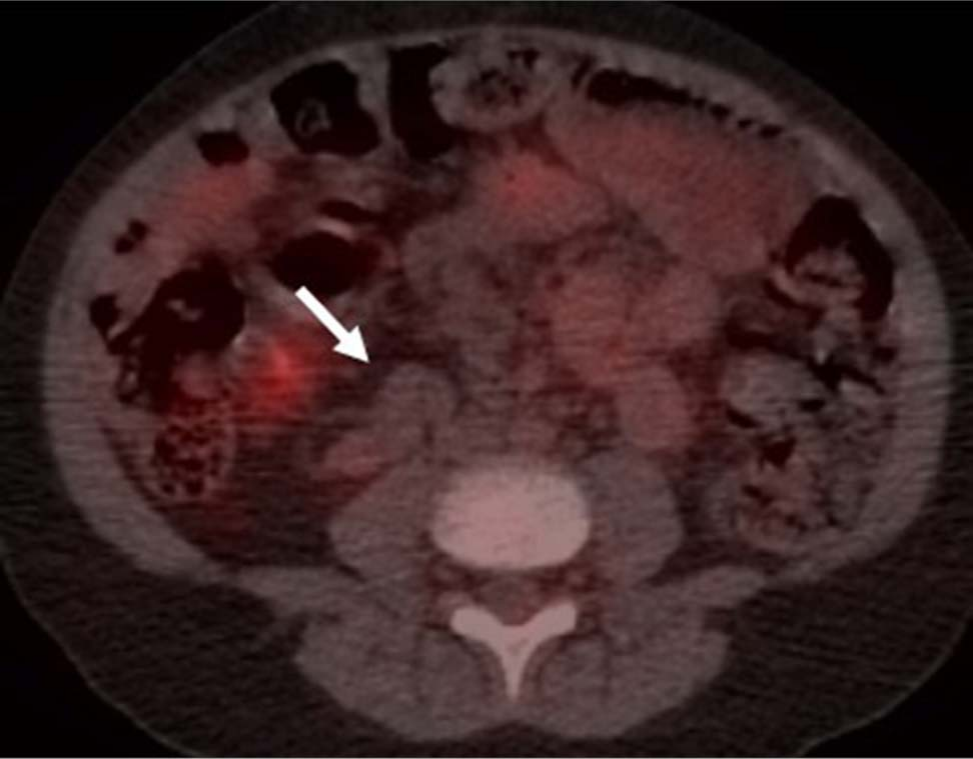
FDG PET/CT scan demonstrated interval-resolved FDG activity of the retroperitoneal lesions 6 weeks after dose reduction of immunosuppression therapy. CT, computed tomography; PET, positron emission tomography; SUV, standardized uptake value.
